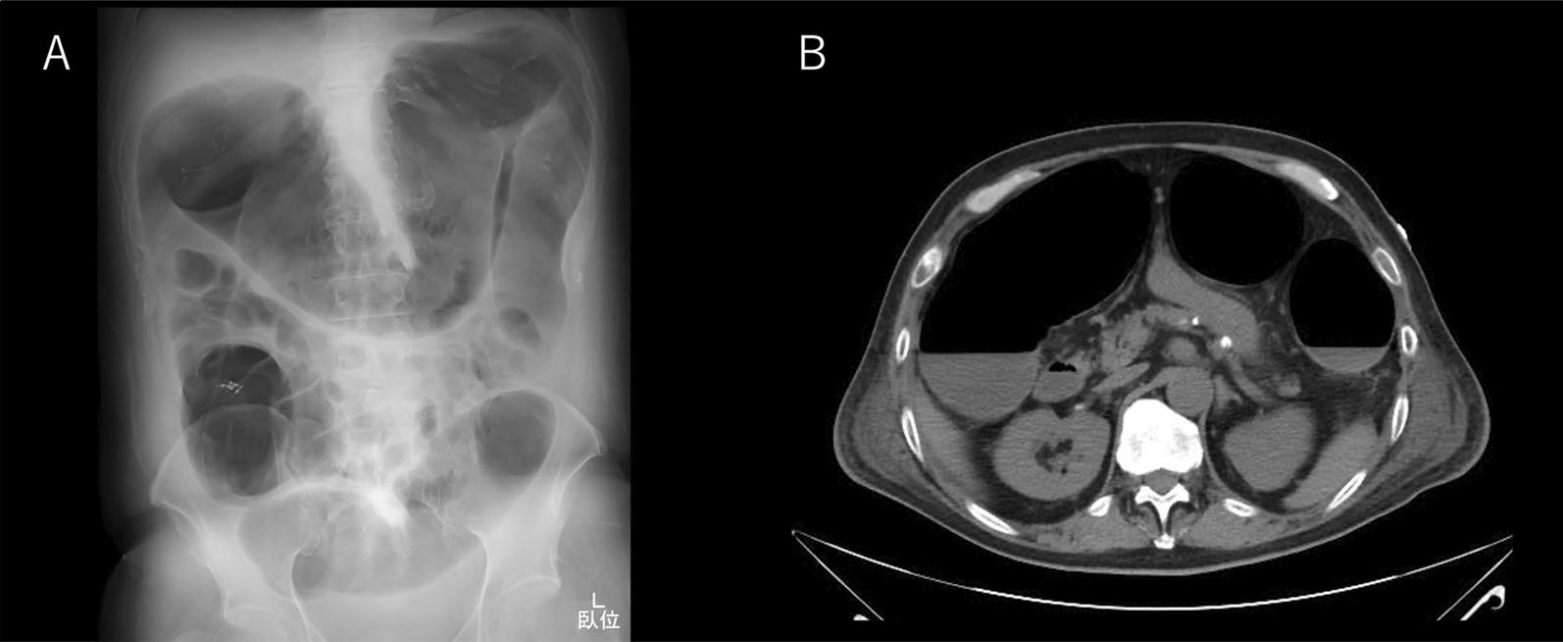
Abdominal radiography (A) and contrast-enhanced computed tomography (CT) scan (B) obtained at admission: an abdominal radiograph showing a dilated ahaustral transverse colon. Abdominal CT shows diffuse dilatation of the transverse colon up to 80 mm in diameter
